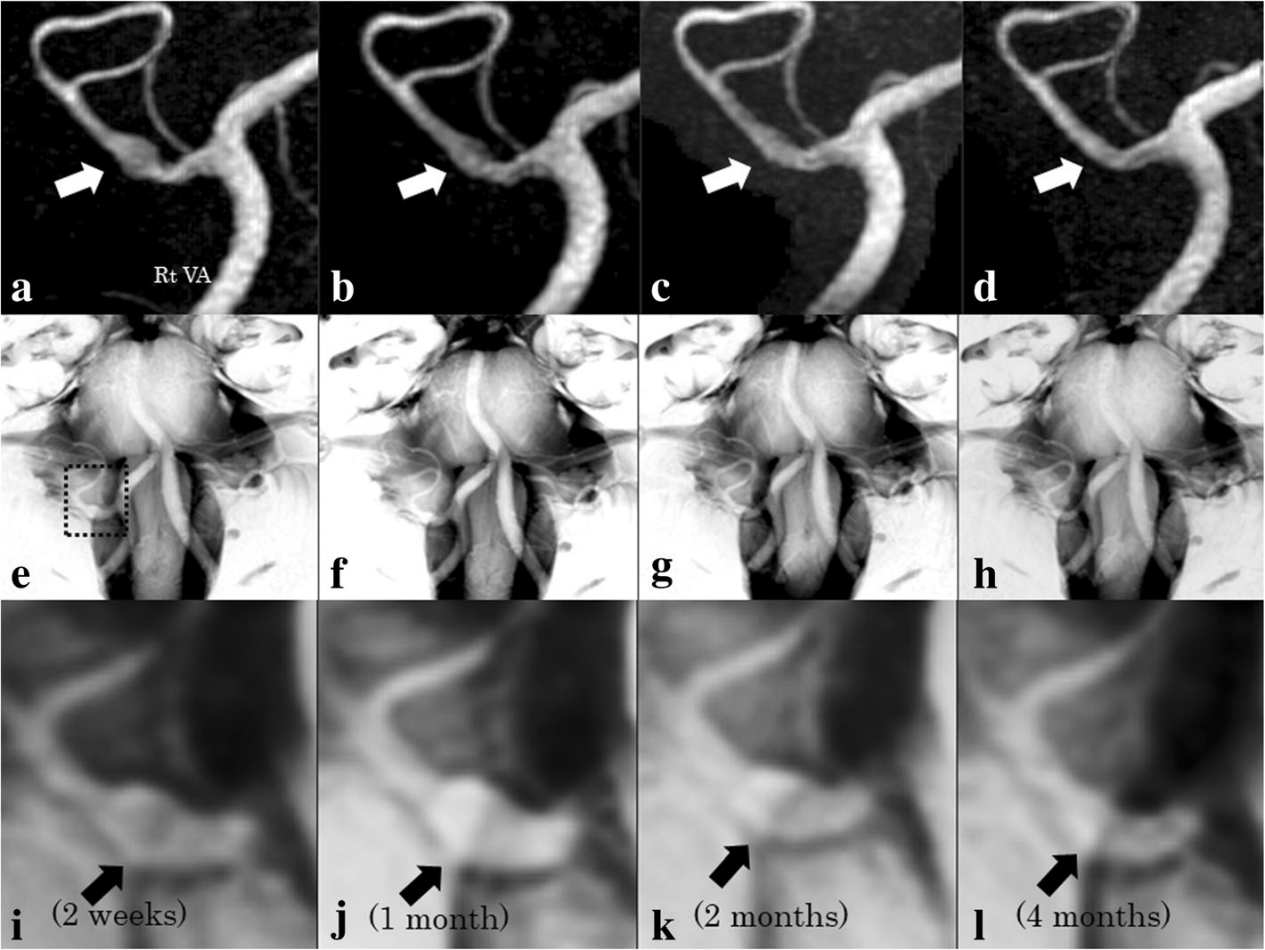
MRA (MIP) in upper raw (a-d), BPAS in middle raw (e-h) and BPAS zooming in the affected PICA in lower raw (i-l). From the left column to the right column, the data of 2 weeks after the onset (a, e and i), 1 month (b, f and j), 2 months (c, g and k) and 4 months (d, h and l) are lined. Chronological changes of PICA inner diameter (MIP) and outer diameter (BPAS) are shown. MIPs show that the inner diameter of the right PICA grows maximally on the 14th day and are gradually downsizing. These MRI findings show the inner diameter reduction precedes the outer diameter’s shrinkage
